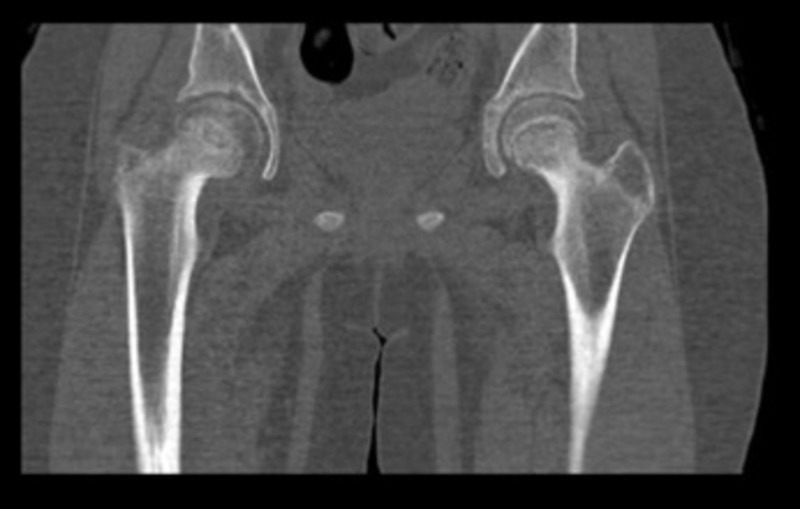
Coronal CT scan The CT scan showed a moderate bilateral posterior slippage of femoral heads; the right and left femoral head-neck angles measured 60 and 52 degrees, respectively. CT: computed tomography
